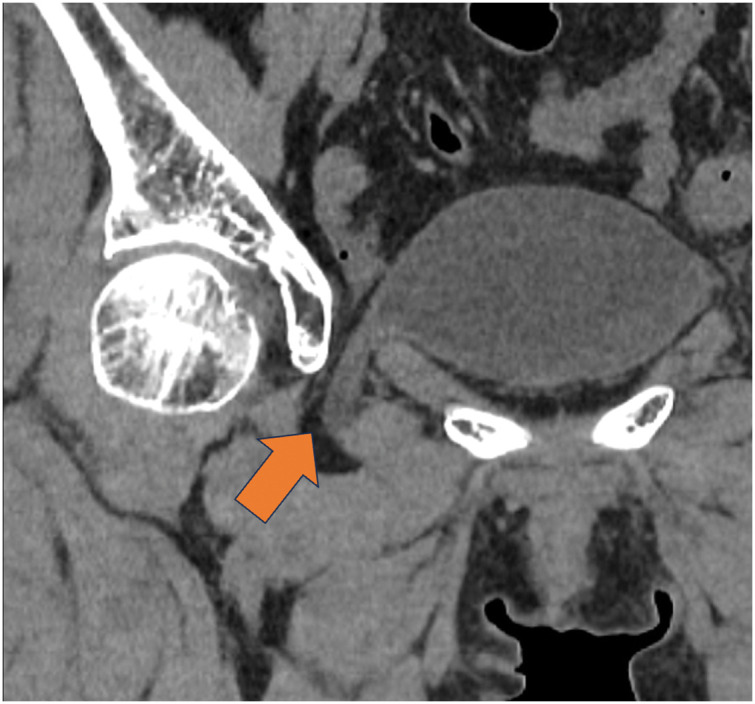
The CT scan performed on the following day at the referring hospital. Arrow: bladder herniation through the obturator foramen.
